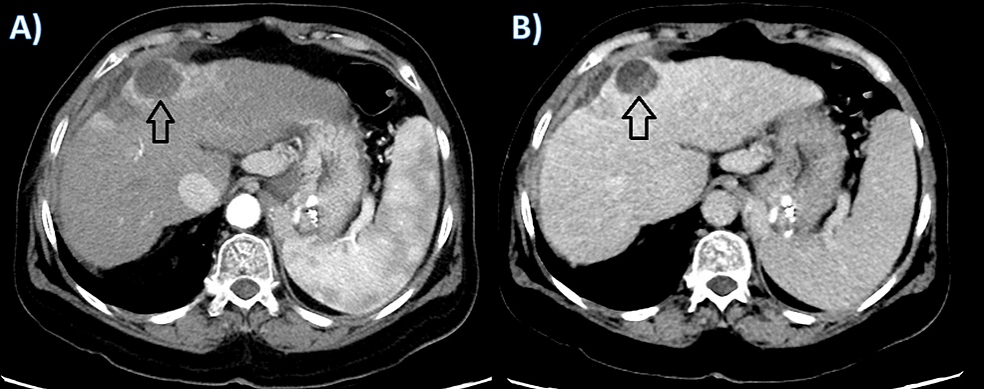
A: arterial phase; B: portovenous phase. A follow-up contrast enhanced axial CT scan image after three months in showing significant reduction in size to 2.4 cm x 2.5 cm and enhancement of the treated tumor in segment IV.
